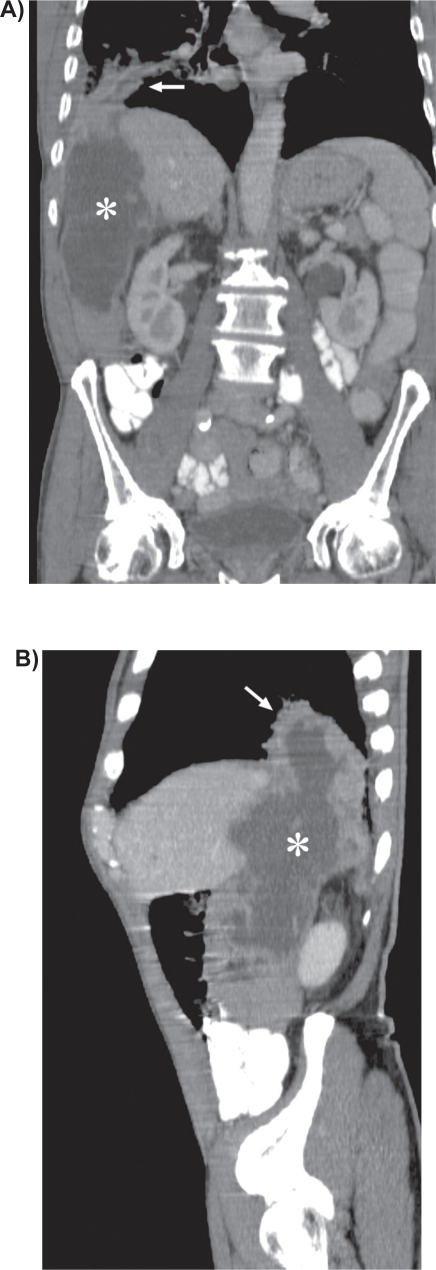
Coronal A) and sagittal B) reformatted CT images reveal the large cystic mass (asterix) extending from the region of the appendix retroperitoneally behind the liver into the right lower lobe (arrow).
